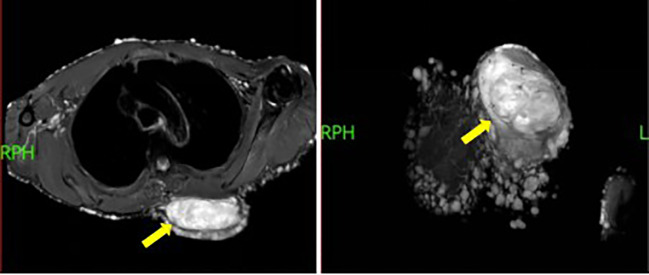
MRI contrast-enhanced scan showing nodular and mass-like shadows. The arrow indicates a mass on the left side of the chest and back.
